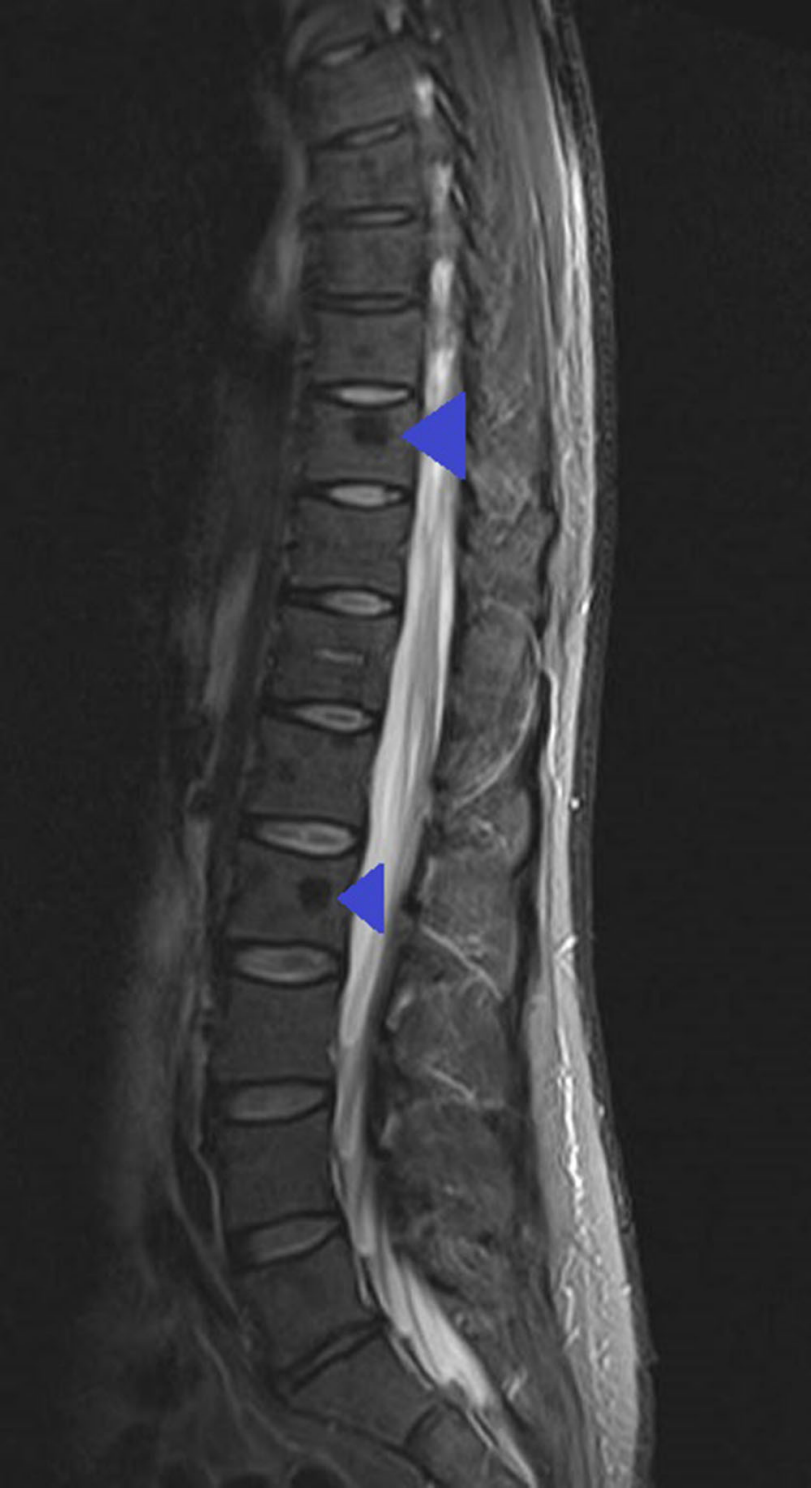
Magnetic resonance imaging (MRI) thoracic and lumbar spine demonstrating low signal lesions in the T10 and L2 vertebral bodies
